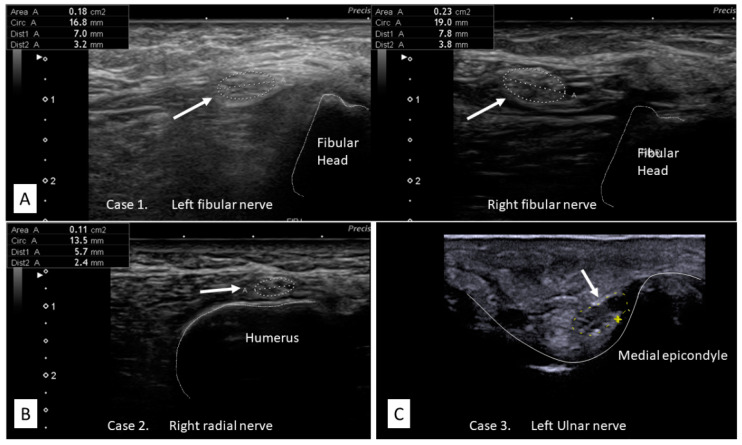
Nerve ultrasound images. (A) Case 1: The bilateral fibular nerve shows focal swelling with increased CSA between the fibular head and the popliteal fossa in both legs, suggesting a compression neuropathy at the fibular head (left: 18 mm^2^, right: 23 mm^2^) *. (B) Case 2: The left radial nerve shows focal swelling with increased CSA (11 mm^2^) *, suggesting compression neuropathy at the spiral groove. (C) Case 3: The left ulnar nerve shows focal swelling with increased CSA (13 mm^2^) *, suggesting a compression neuropathy at the cubital tunnel. CSA, cross-sectional area. * Normal values for CSA at the evaluated sections of our laboratory: the fibular nerve, 12 mm^2^; the radial nerve, 8 mm^2^; the ulnar nerve, 10 mm^2^.
